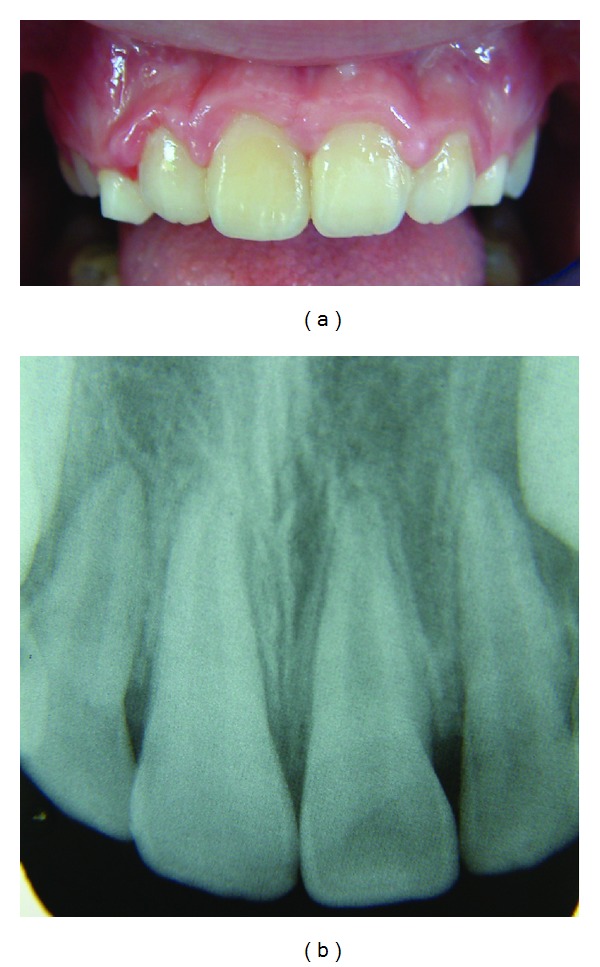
Clinical and radiographic view of the maxillary incisor at 2 years.
